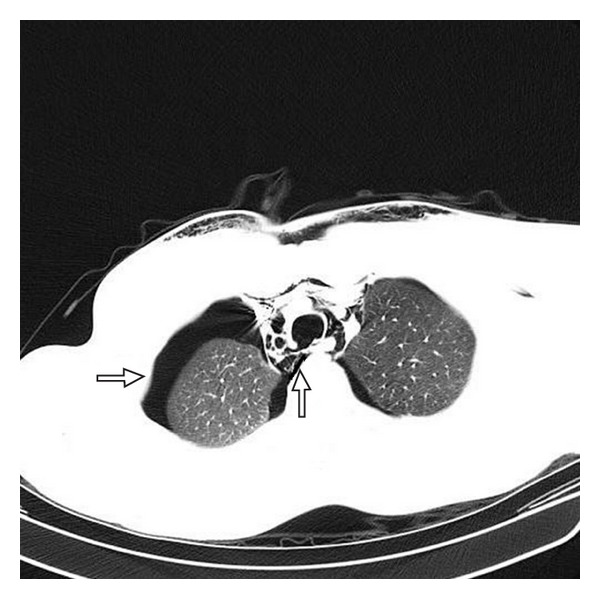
CT chest image depicting tear in the posterior part of the trachea with a right-sided pneumothorax.
